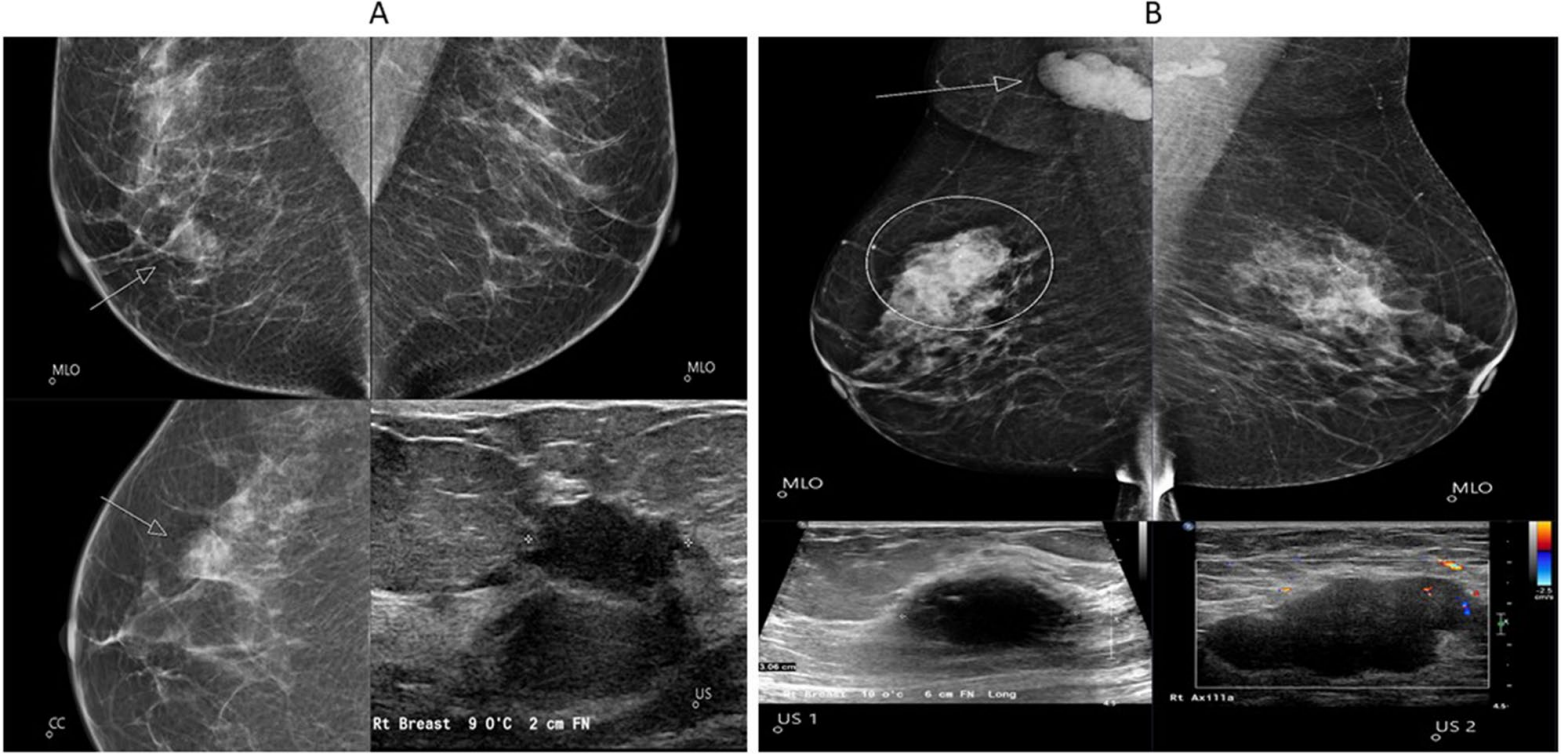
Examples of cancers missed by the AI system. A A 15-mm-large invasive ductal cancer (grade 2) with a soft density appearance (arrows) in both views lacks distinct margins. AI risk score of 5/10 and detected by one of two radiologists. MLO, mediolateral oblique view; CC, craniocaudal view, and ultrasound detail. B A 29-mm-large invasive ductal cancer (grade 3) (US1) is masked within the dense asymmetric fibroglandular tissue in the right upper outer quadrant (circle) with significant axillary lymphadenopathy (arrow and US 2). Both radiologists recalled this case—AI algorithm fails to recognise the context of the lymph node and provides low-risk score of 7. MLO, mediolateral oblique view; CC, craniocaudal view and US, ultrasound details
